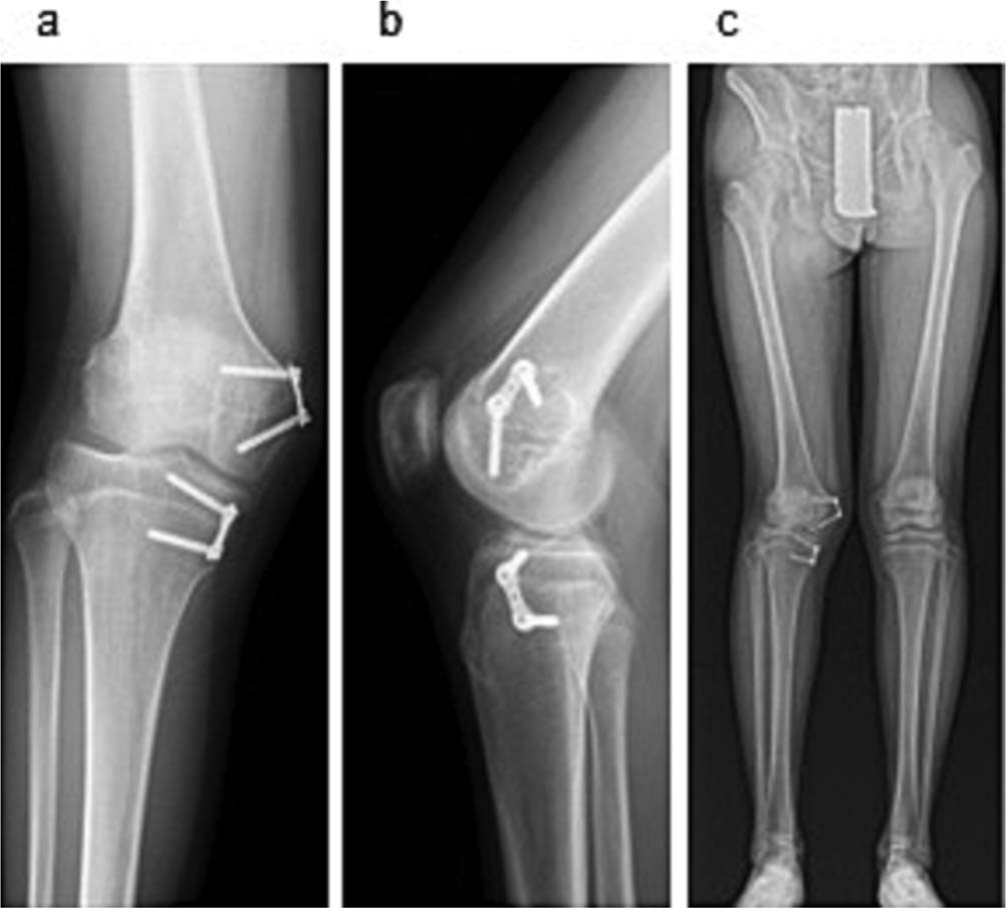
Radiographic findings at 24-month follow-up. (a) Anteroposterior radiograph of the right knee. (b) Lateral radiograph of the right knee. (c) Full-length standing anteroposterior radiograph of the lower extremities.
